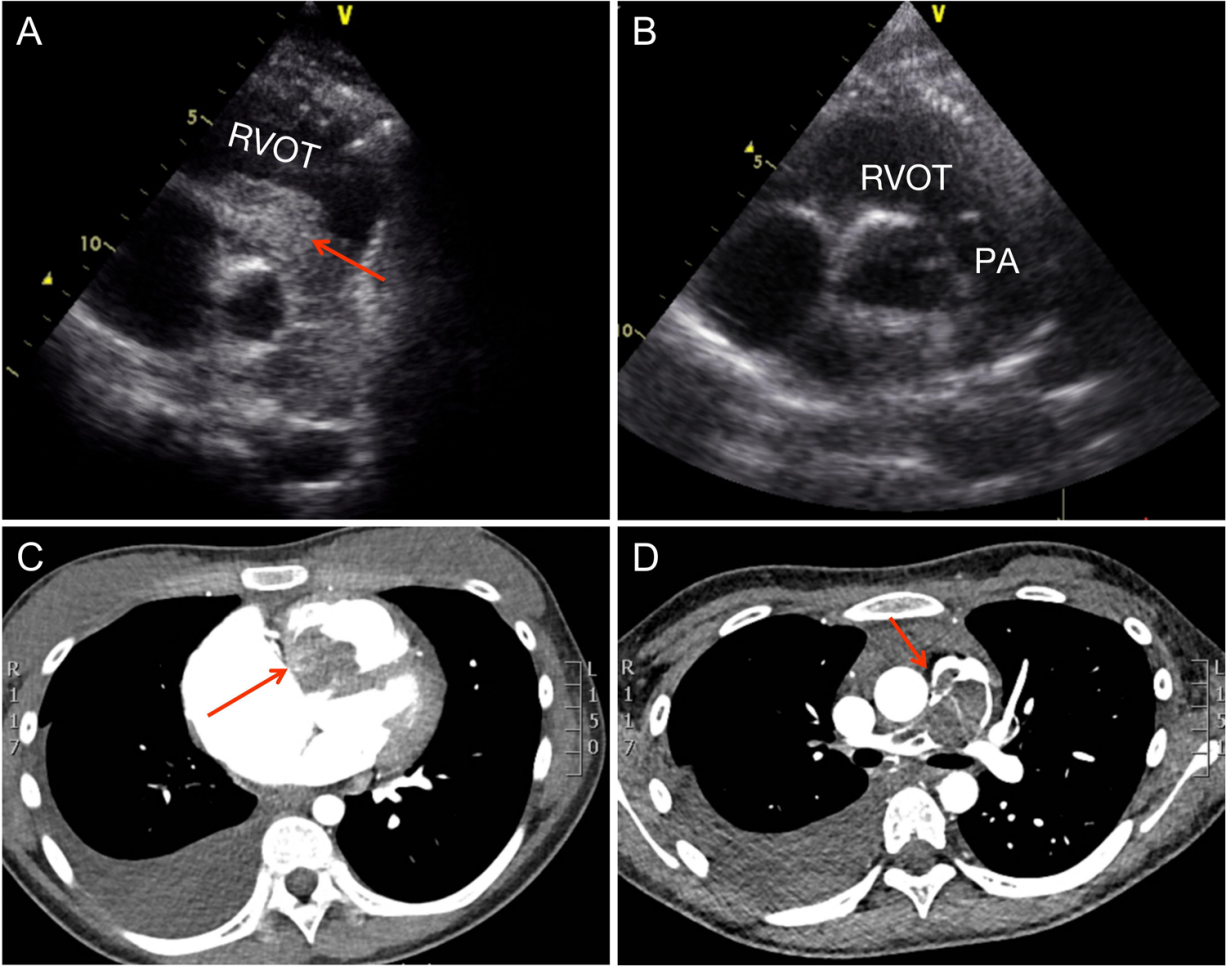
Preoperative and postoperative examination. (A) Preoperative parasternal short-axis view at the level of the aortic valve showing the obstructed right ventricular outflow tract and pulmonary trunk. (B) Postoperative parasternal short-axis view at the level of the aortic valve showing the unobstructed right ventricular outflow tract and pulmonary trunk. (C) Computed tomography reveals the right ventricular outflow tract. (D) Computed tomography reveals the pulmonary trunk and right pulmonary artery. RVOT, right ventricular outflow tract; PA, pulmonary artery.
